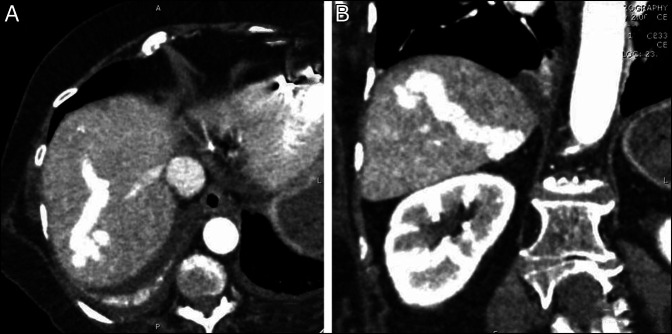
(A) Axial contrast-enhanced computed tomography demonstrating segment 7 large intrahepatic portovenous shunt and (B) coronal contrast-enhanced computed tomography demonstrating segment 5 large intrahepatic portovenous shunt.
